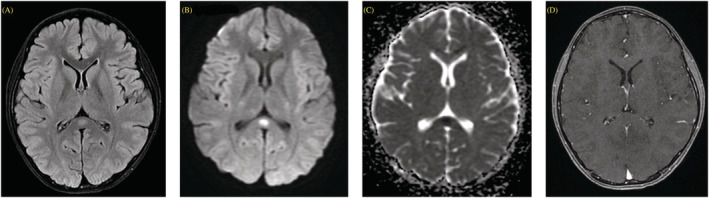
Brain magnetic resonance imaging (MRI) of mild encephalitis/encephalopathy with reversible splenial lesion (MERS) (Case No. 3). (A) Axial longitudinal relaxation time‐weighted imaging (T1WI), (B) transverse relaxation time‐weighted imaging (T2WI)/fluid‐attenuated inversion recovery (FLAIR), (C) diffusion‐weighted imaging (DWI), and (D) apparent diffusion coefficient (ADC) show circumscribed oval‐shaped non‐enhancing T2/FLAIR hyperintense lesion with diffusion restriction involving splenium of the corpus callosum. This finding is typical for cytotoxic lesion of the corpus callosum (CLOCC).
